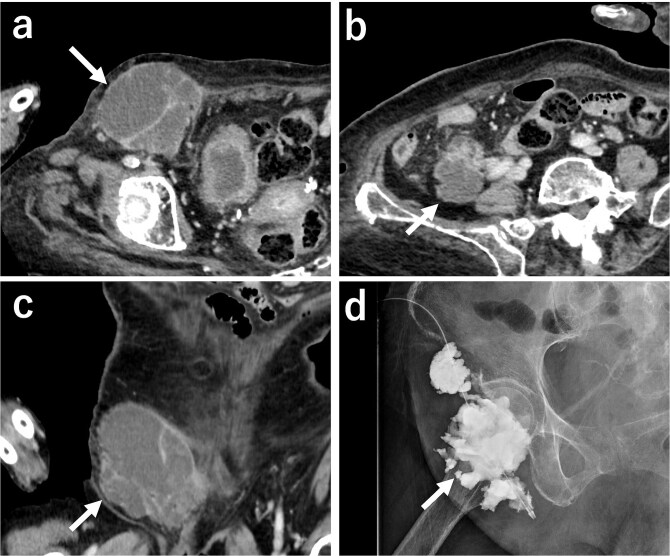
Postoperative imaging. (a–c) Abdominal CT 4 days after mastectomy. The fluid collection can be seen in the right groin area and around the cecum. The collection had formed outside the hernia sac at the groin. (d) Contrast imaging of the abscess. No communication between the abscess and the intestinal tract is observed.
